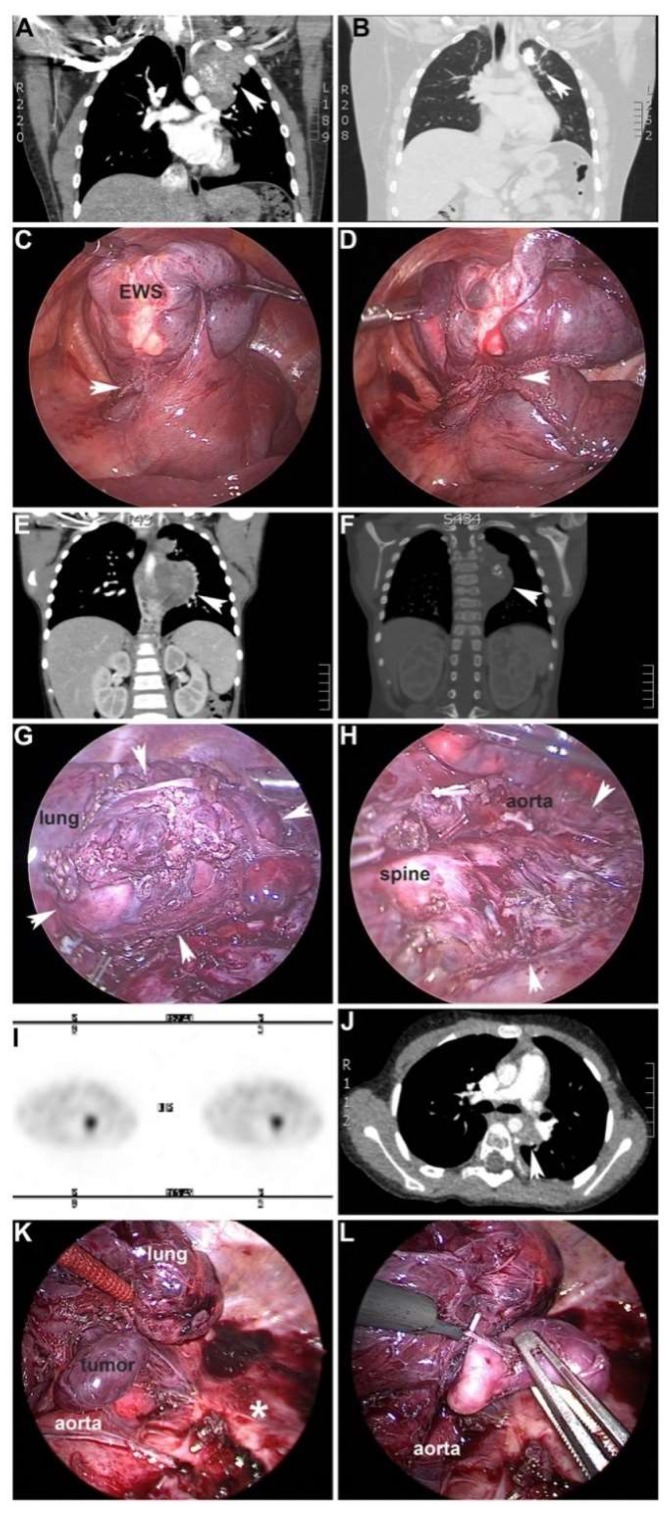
Minimally invasive surgery (MIS) resection of thoracic pediatric embryonal tumors. (A–C) Adolescent male who was diagnosed with a primary Ewing sarcoma (EWS) of the left upper pulmonary lobe (A; arrowhead). Marked regression of primary lesion was observed after neoadjuvant therapy (B; calcified nodule and arrowhead). (C) Thoracoscopic view of superior segment of left upper lobe and mass (EWS). Arrowhead denotes staple line after dividing segmental artery to mass. (D) Completing the segmentectomy with a linear stapler. Arrowhead denotes resection staple line. (E–H) Four-year-old girl who presented with a large left-sided thoracic neuroblastoma (NBL). (E,F) Mass before and after neoadjuvant therapy (arrowhead). (G,H) Thoracoscopic resection of large thoracic NBL. (G) Borders of the mass after mobilization are depicted with arrowheads. Collapsed lung is labeled. Note the profound angiogenic nature of NBL and desmoplastic response after neoadjuvant therapy. (H) Tumor bed after complete resection. Arrowheads depict cephalad and caudal borders of tumor bed. (I–L) Three months after completing therapy, Metaiodobenzylguanidine (MIBG)-avidity of a hilar lymph node persisted (I; dark spot). Arrowhead denotes mass on CT scan (J). (K,L) Repeat thoracoscopic approach to resect hilar “tumor” 12 months after initial operation. Lung and aorta are labeled. Asterisk denotes primary tumor bed free of local relapse (K). (L) Hook cautery dissection of dumbbell-shaped hilar mass in atraumatic grasper.
